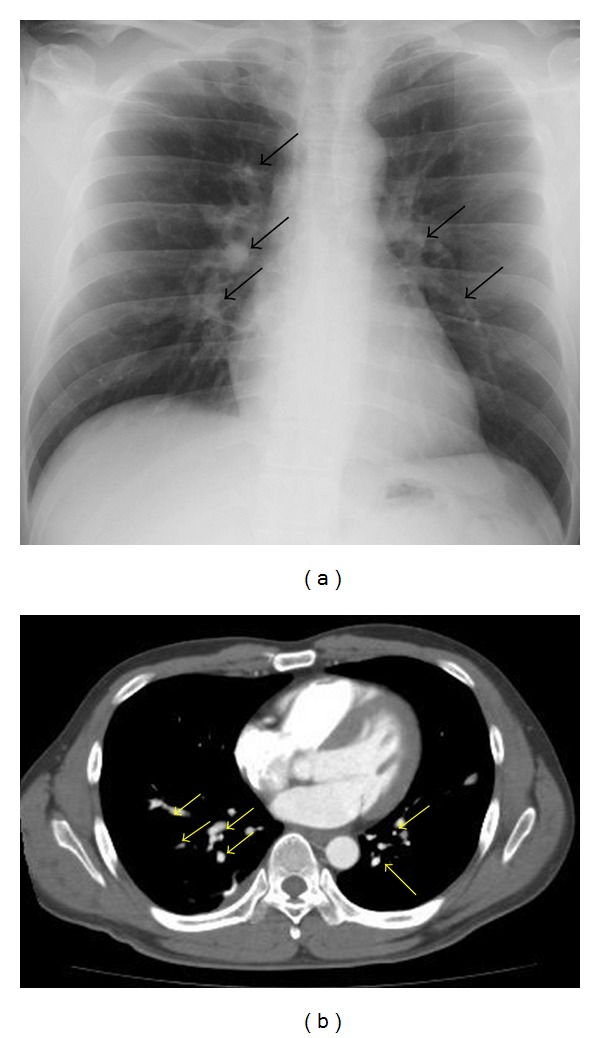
Chest radiograph (a) and computed tomography (CT) (b) reveal multiple tumors in both lobes. Arrows represent meningioma metastases.
